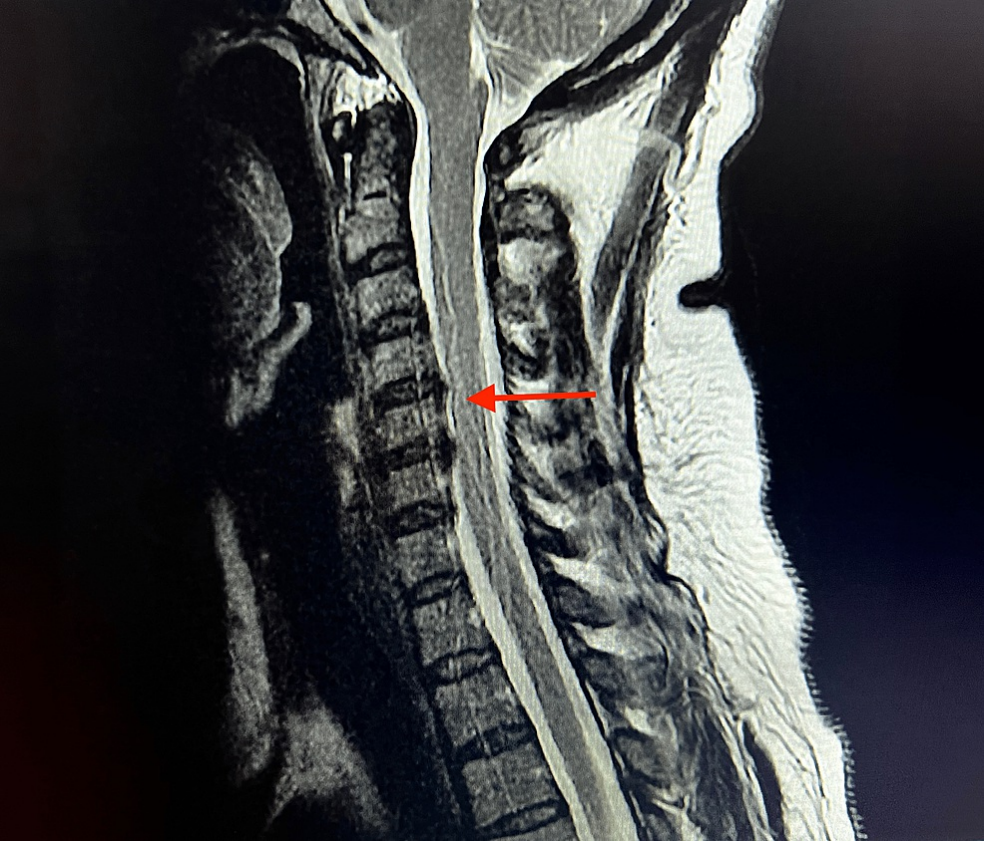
MRI of the cervical spine with and without contrast: mild spondylosis throughout the cervical spine and mild broad-based disc bulging (red arrow) at C5-C6 without any significant neural foraminal narrowing
